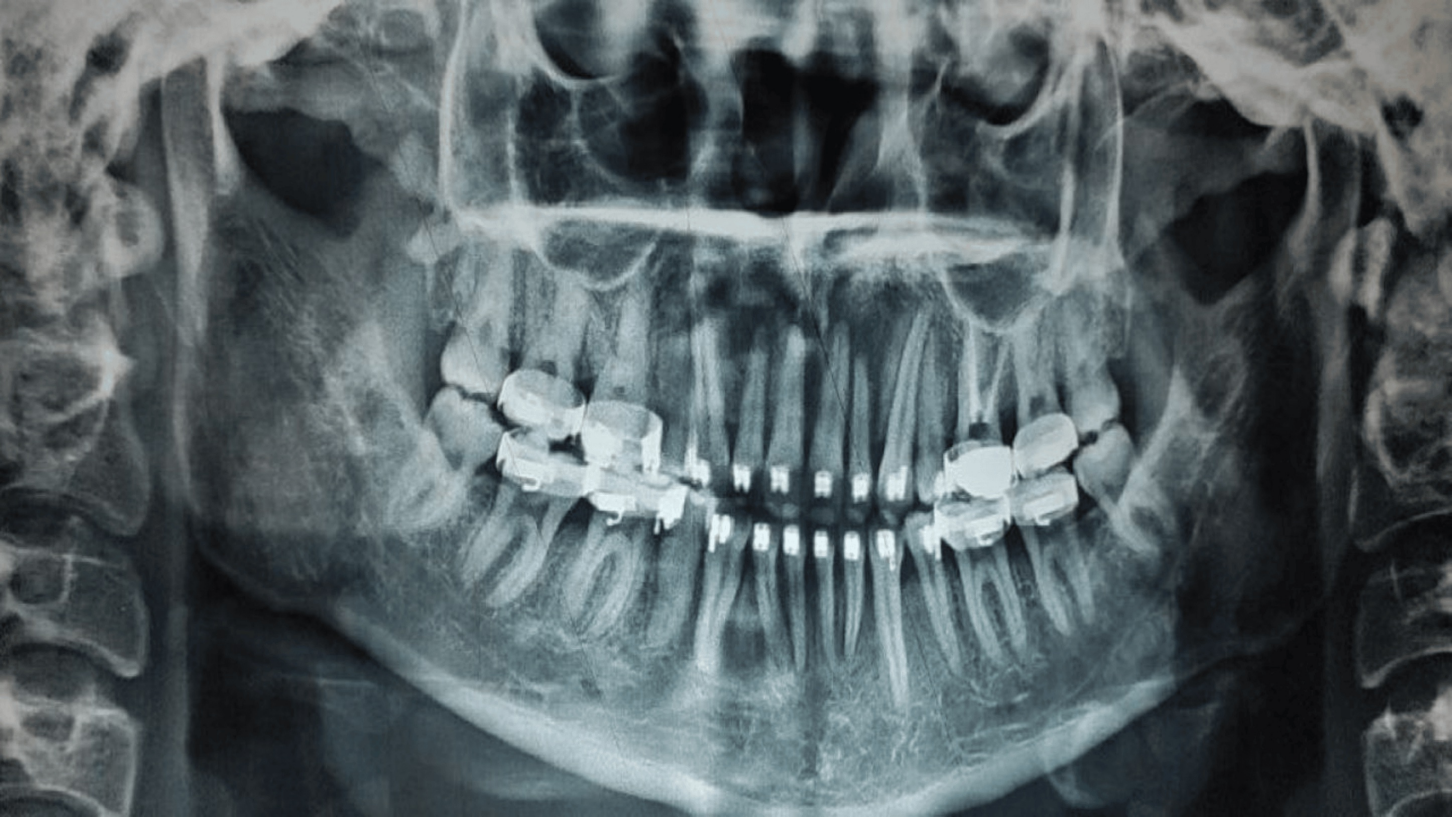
Post-treatment orthopantomogram.
